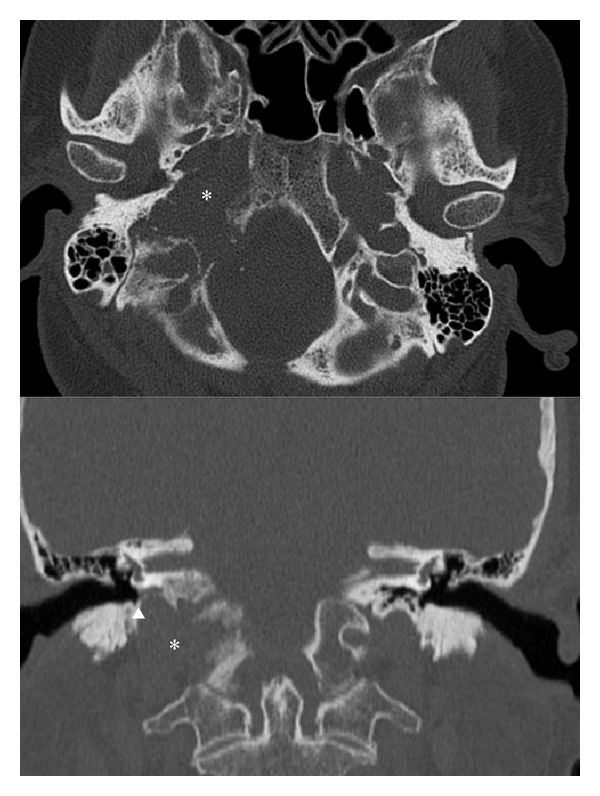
Axial and coronal nonenhanced CT scan reformations using bone reconstruction algorithm demonstrates an osteolytic skull base lesion centered within the right jugular foramen (∗) the lesion exhibits irregular margins with preservation of the ipsilateral hypotympanic sigmoid plate (arrowhead) and without macroscopic extension into the ipsilateral middle ear.
